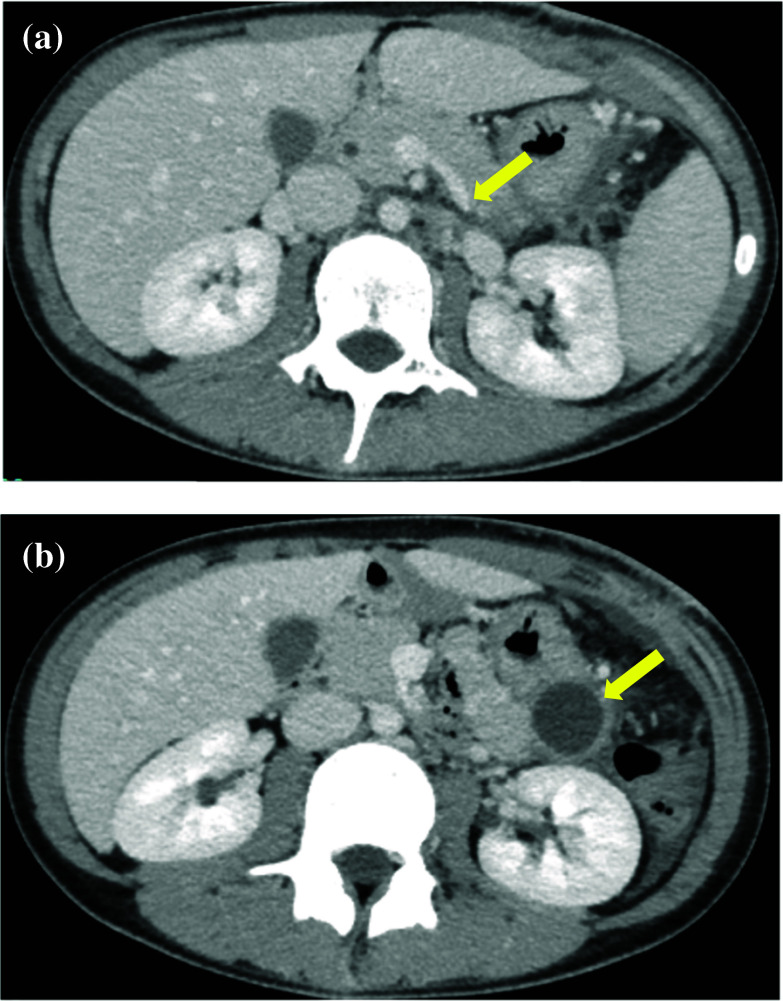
a Enhanced CT. Splenic vein stenosis remained at POD 13 (yellow arrow). b Enhanced CT. A cyst of POPF revealed at the stump of pancreas tail at POD 13 (yellow arrow)
